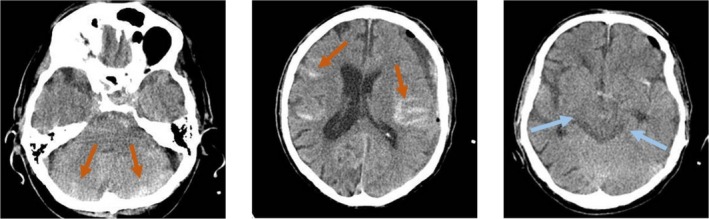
Postoperative Brain CT scan. Left image: Cerebellar subarachnoid hemorrhage. Middle image: Hemispheric subarachnoid hemorrhage. Right image: Basal cisterns are bilaterally symmetrically visible.
